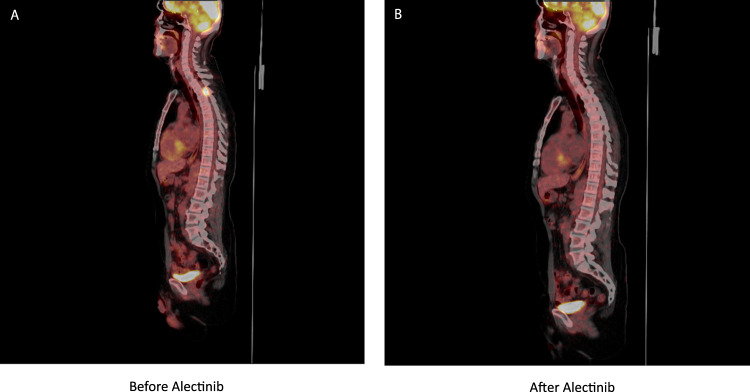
PET Scan results. A Before initiation of Alectinib. Foci of increased uptake in a mediastinal lymph node (max SUV 6.3), retrocaval lymph node (max SUV 10.6), right T3/T4 intervertebral foramen (max SUV 14) on PET-CT. B Complete response after 2 months of alectinib. Complete resolution of prior noted upper thoracic spine lesion and upper abdominal pericaval nodule.
